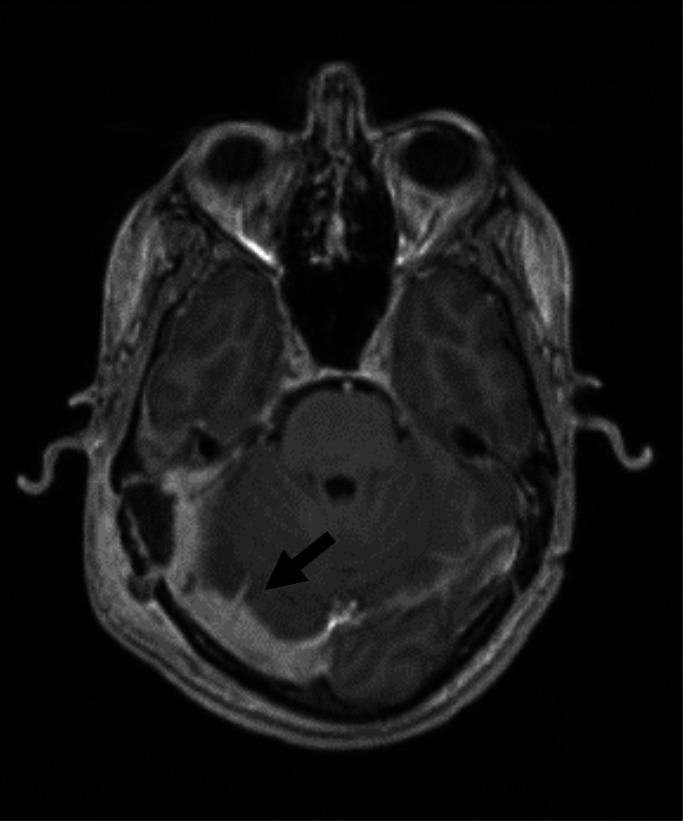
Magnetic resonance image (T1-weighted sequence): Thrombosis of right sigmoid sinus. Inflammatory thickening of dura-mater with involvement of right temporal basal region. Oedema and contrast enhancement of retroauricular and occipital soft tissues.
